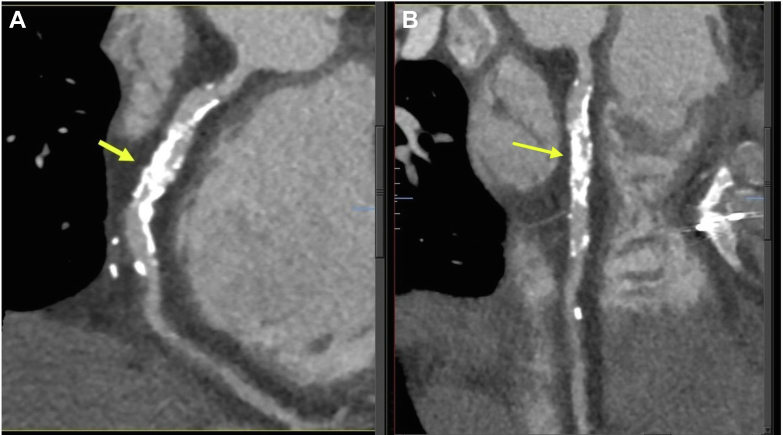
Computed Tomography Coronary Angiography Calcific stenoses of the right coronary artery: (A) left anterior oblique view and (B) longitudinal view.
